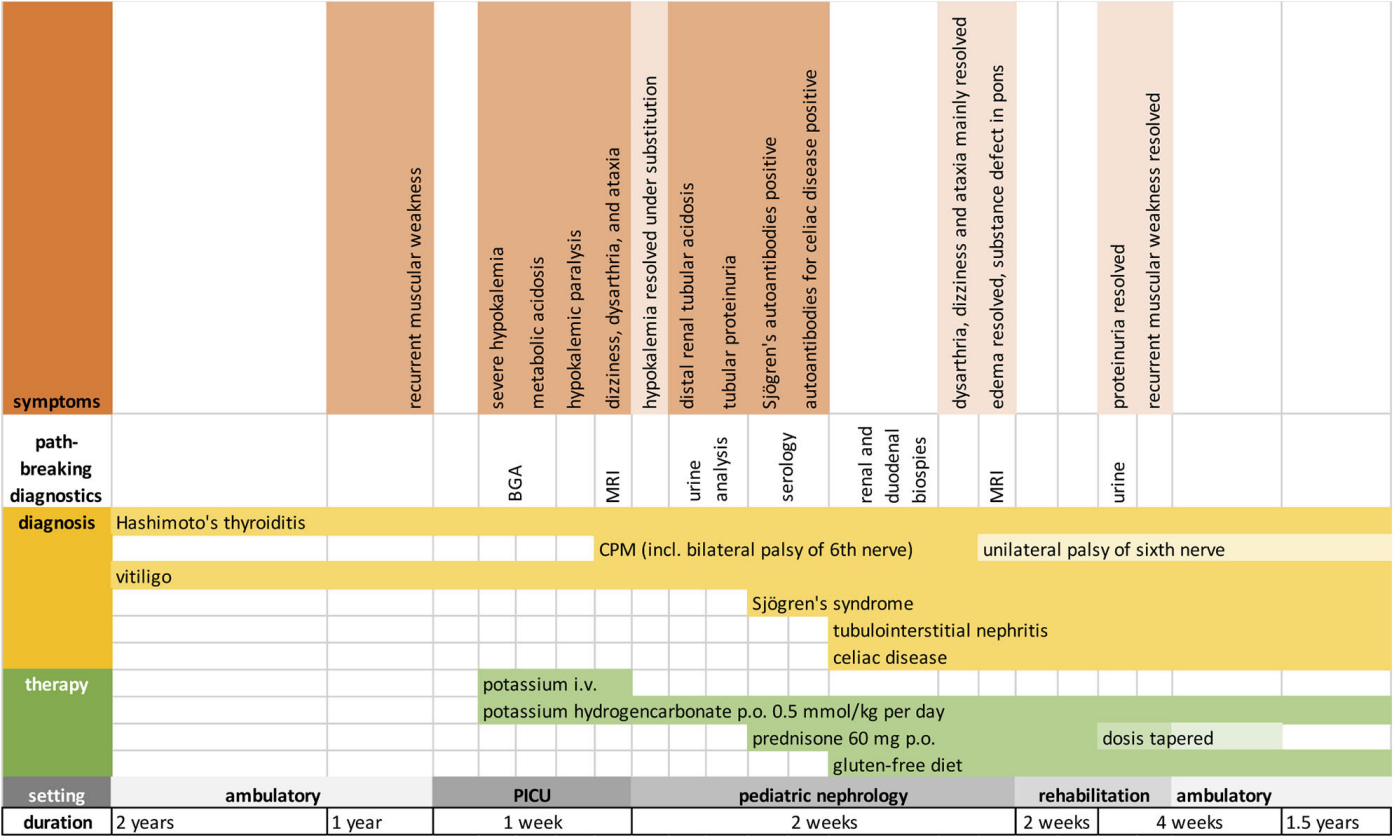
Timeline showing the chronological order of symptoms, diagnoses, and treatment. BGA, blood gas analysis; MRI, magnetic resonance imaging.
